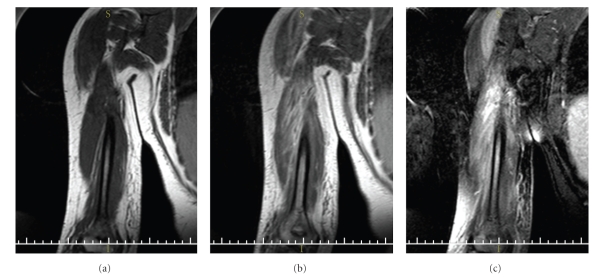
Coronal MRI showing areas of abnormal signal intensity at the inferior aspect of right deltoid muscle and the long and lateral heads of the triceps muscle (b). Please compare to the T1 image without contrast (a). (c) shows postgadolinium enhancement along-with fluid seen in subcutaneous tissue along the lateral aspect of distal right arm.
